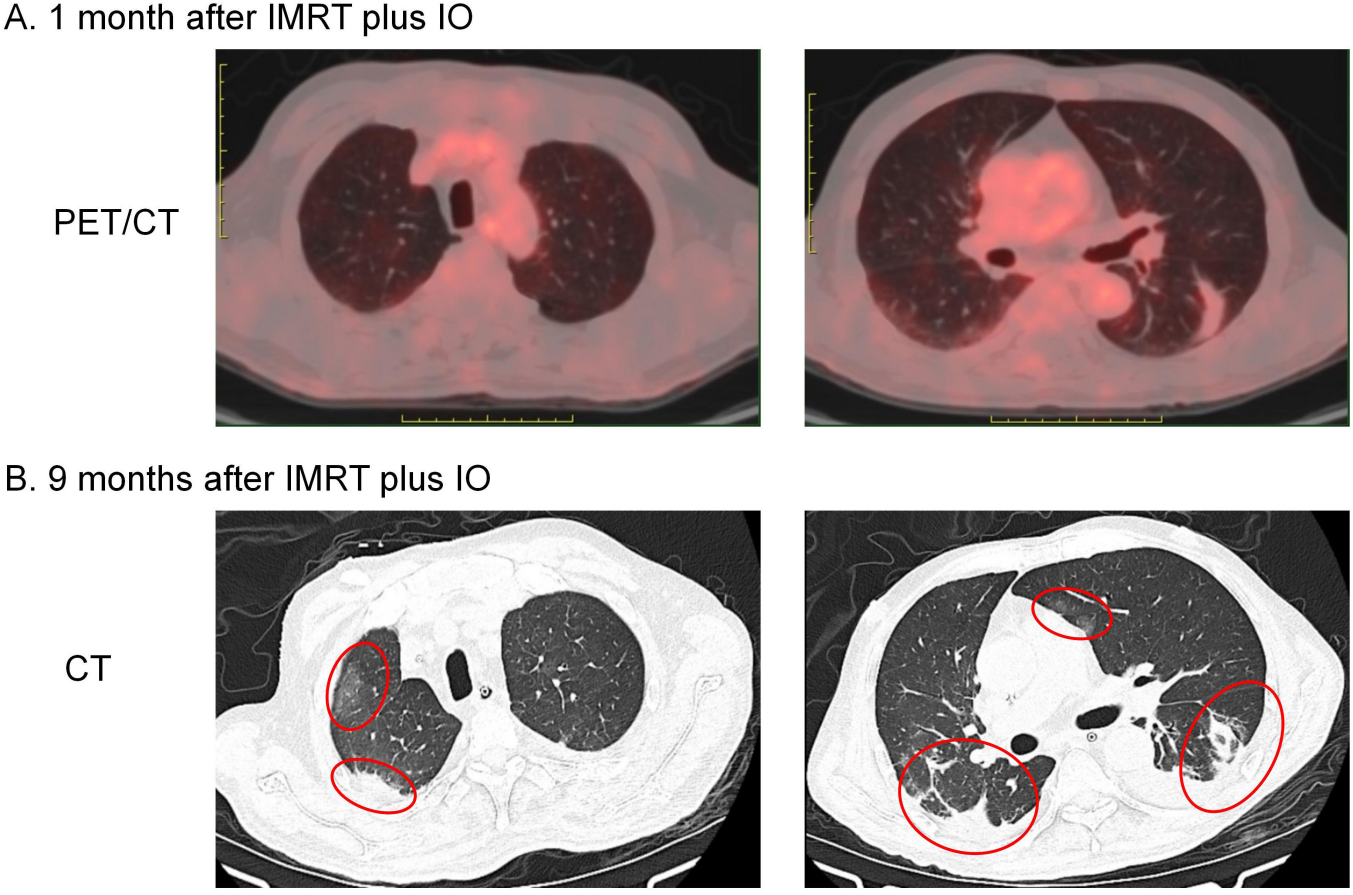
Imaging changes in bilateral lung lesions. (A) PET/CT performed 1 month after IMRT plus IO showed striated lesions without abnormal FDG uptake in the lower lobe of the left lung only. (B) Chest CT performed 9 months after IMRT plus IO showed bilateral pleural effusions with emerging lamellar exudative, semisolid multiple foci underpinned by old interstitial lung changes (indicated by red circles).
